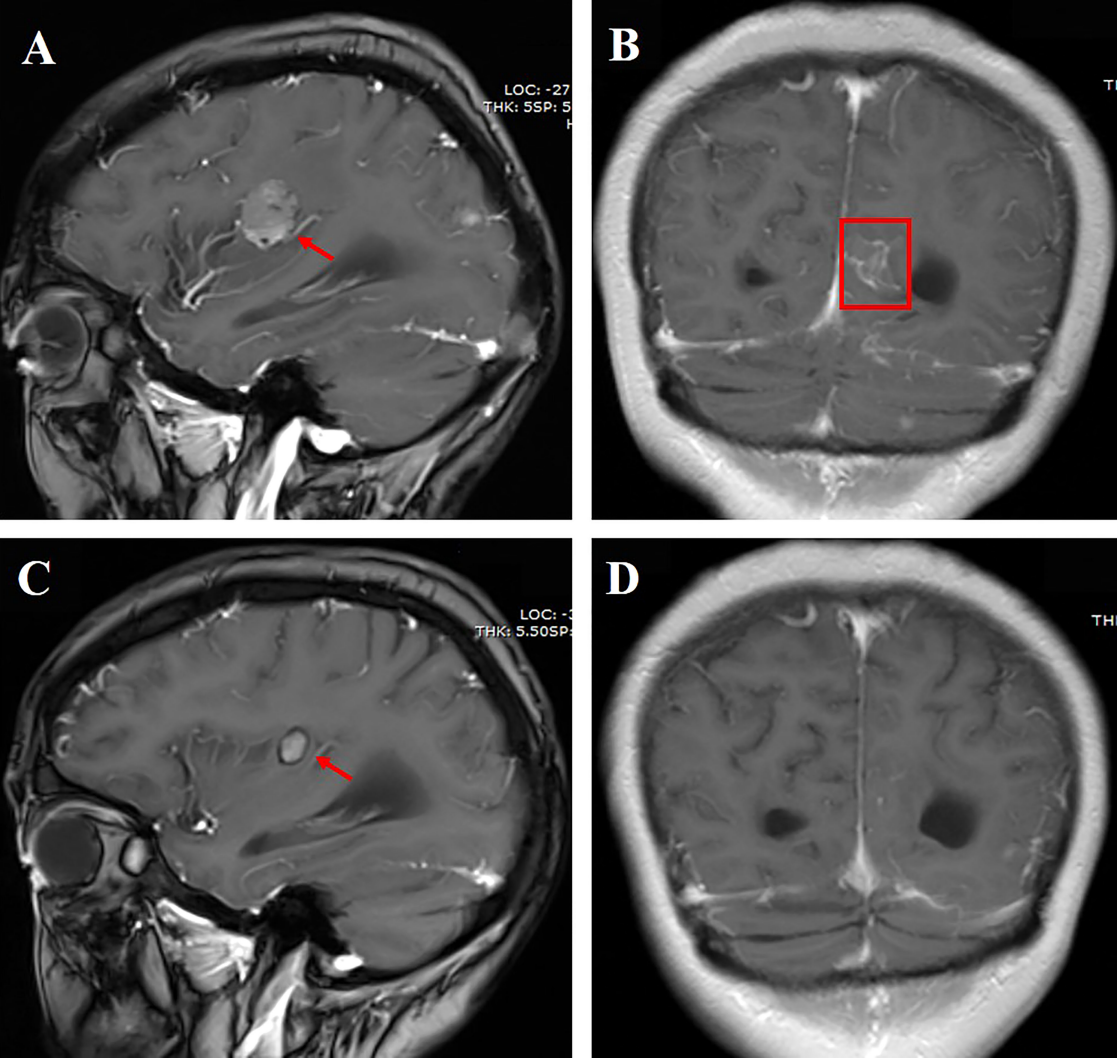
(A) Cranial enhanced MRI showing the right parietal lobe mass measuring approximately 2.1 cm (red arrow); (B) linear enhancement of leptomeninges in the left cerebral hemisphere (red box); (C) the right parietal lobe mass shrank 6 months after aumolertinib plus bevacizumab treatment; (D) linear enhancement of leptomeninges disappeared 6 months after aumolertinib plus bevacizumab treatment.
